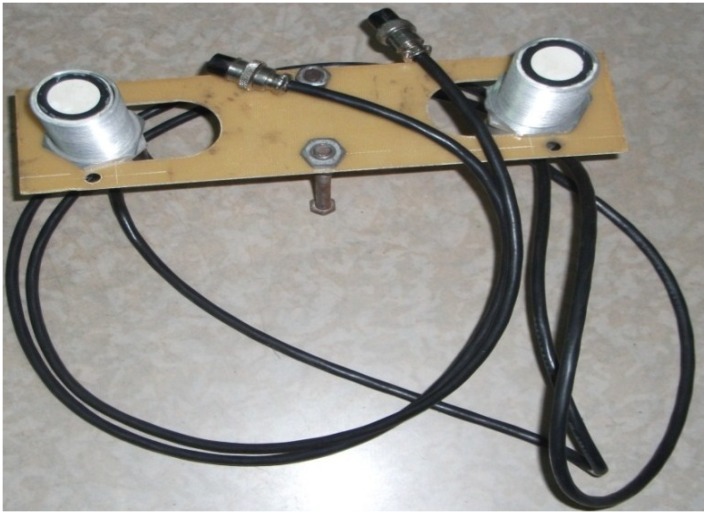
The two parallel ultrasonic sensors fixed on one board.
